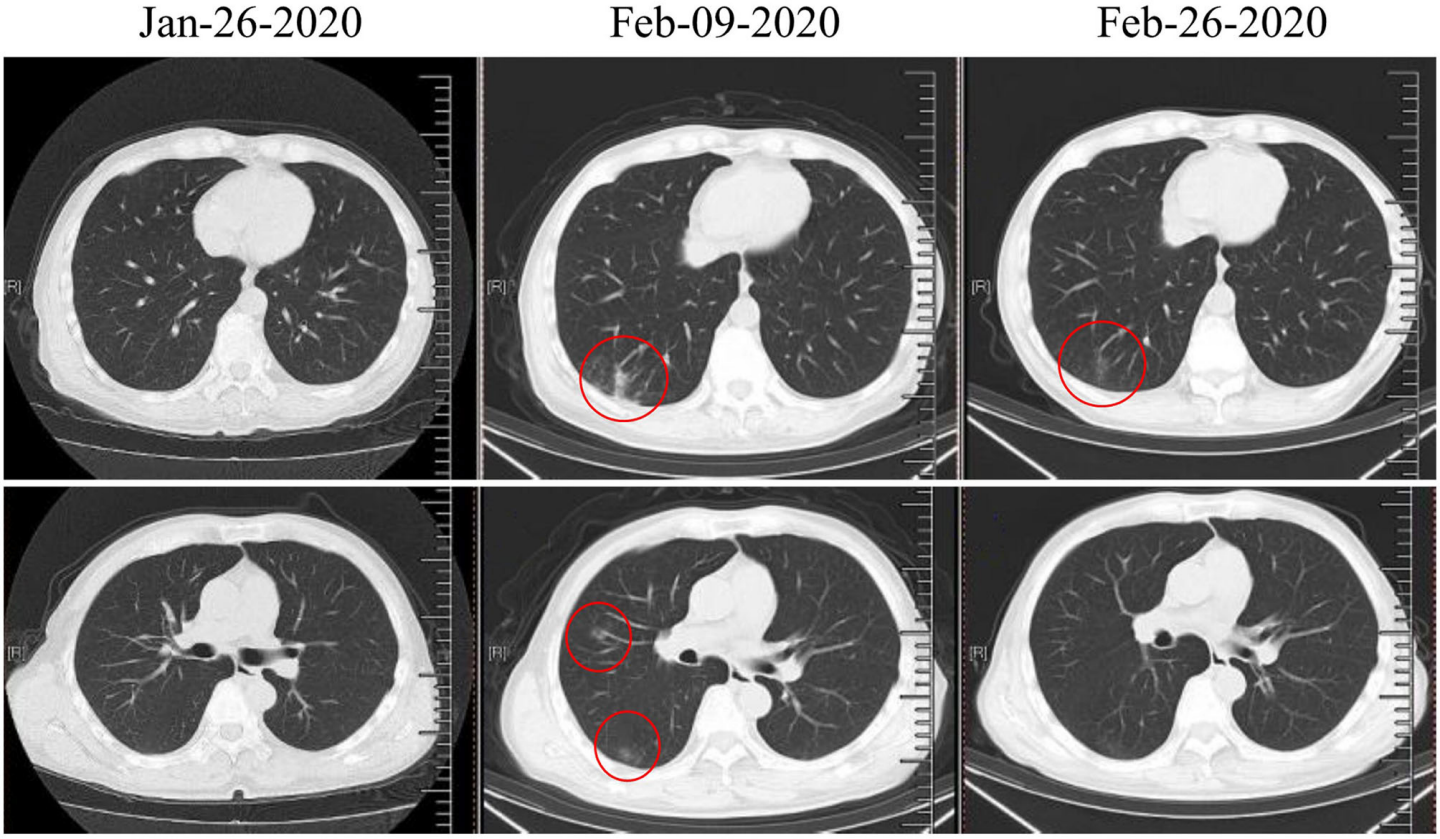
Representative computed tomographic images of three time points. January 26, 2020, day of first fever; February 9, 2020, day of second fever and diagnosis of coronavirus disease (COVID-19); February 26, 2020, 2 days before discharge. Areas of pneumonia are marked with red circles.
